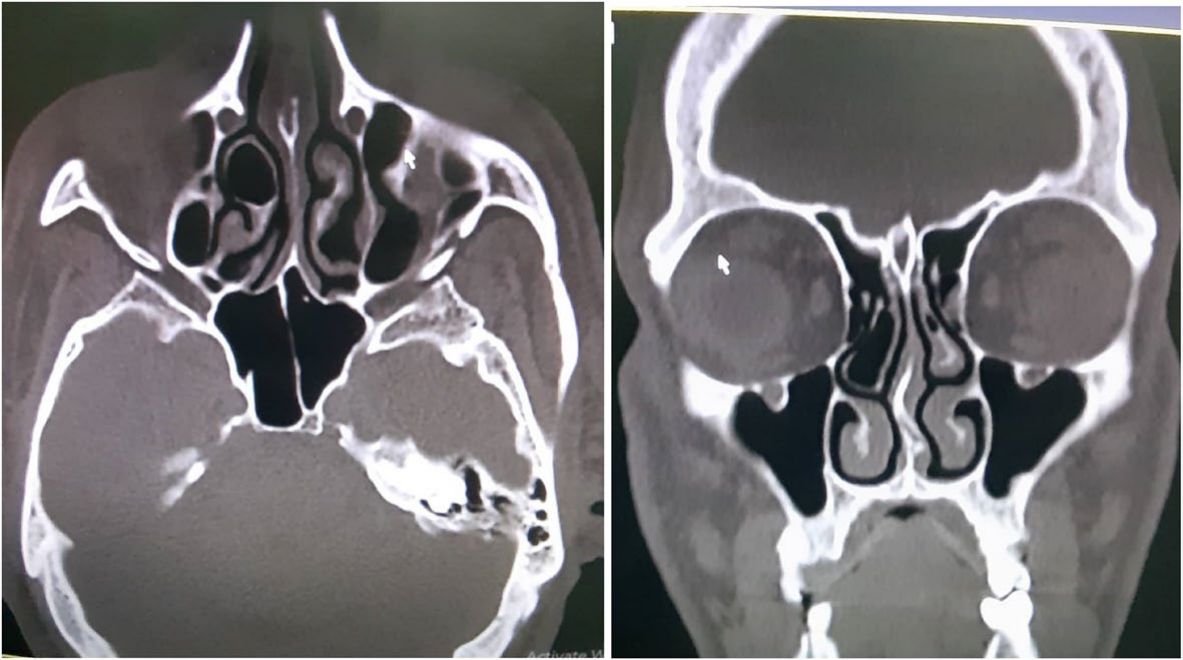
Axial and coronal computed tomography (CT) images showing unilateral concha bullosa involving the right middle turbinate. The pneumatized middle turbinate is clearly outlined, indicating more than 50% vertical height involvement, consistent with the diagnostic threshold for CB
